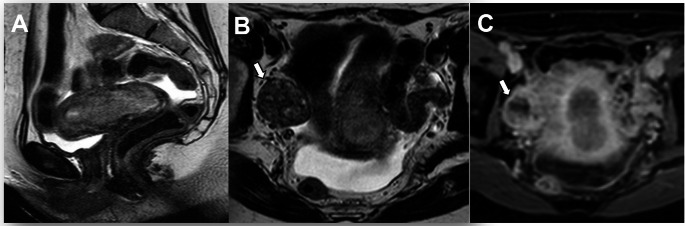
Stage IIIA1 grade 3 endometrioid carcinoma, in a 70-year-old woman. A Sagittal and B axial T2-weighted fast spin-echo (FSE) image (TR/TE: 4500/100 ms; slice thickness: 3 mm) and C) axial contrast-enhanced 3D T1-weighted fat-suppressed LAVA sequence (TR/TE: 4.2/2.1 ms; slice thickness: 2 mm; post-gadolinium, delayed phase at 4 min) show a large endometrial mass distending the uterine cavity with myometrial invasion, extending into the cervical channel with stromal invasion. On axial T2WI and on dynamic contrast-enhanced images a right parauterine heterogeneous mass is demonstrated (arrows). Right ovarian metastasis was confirmed by pathology
